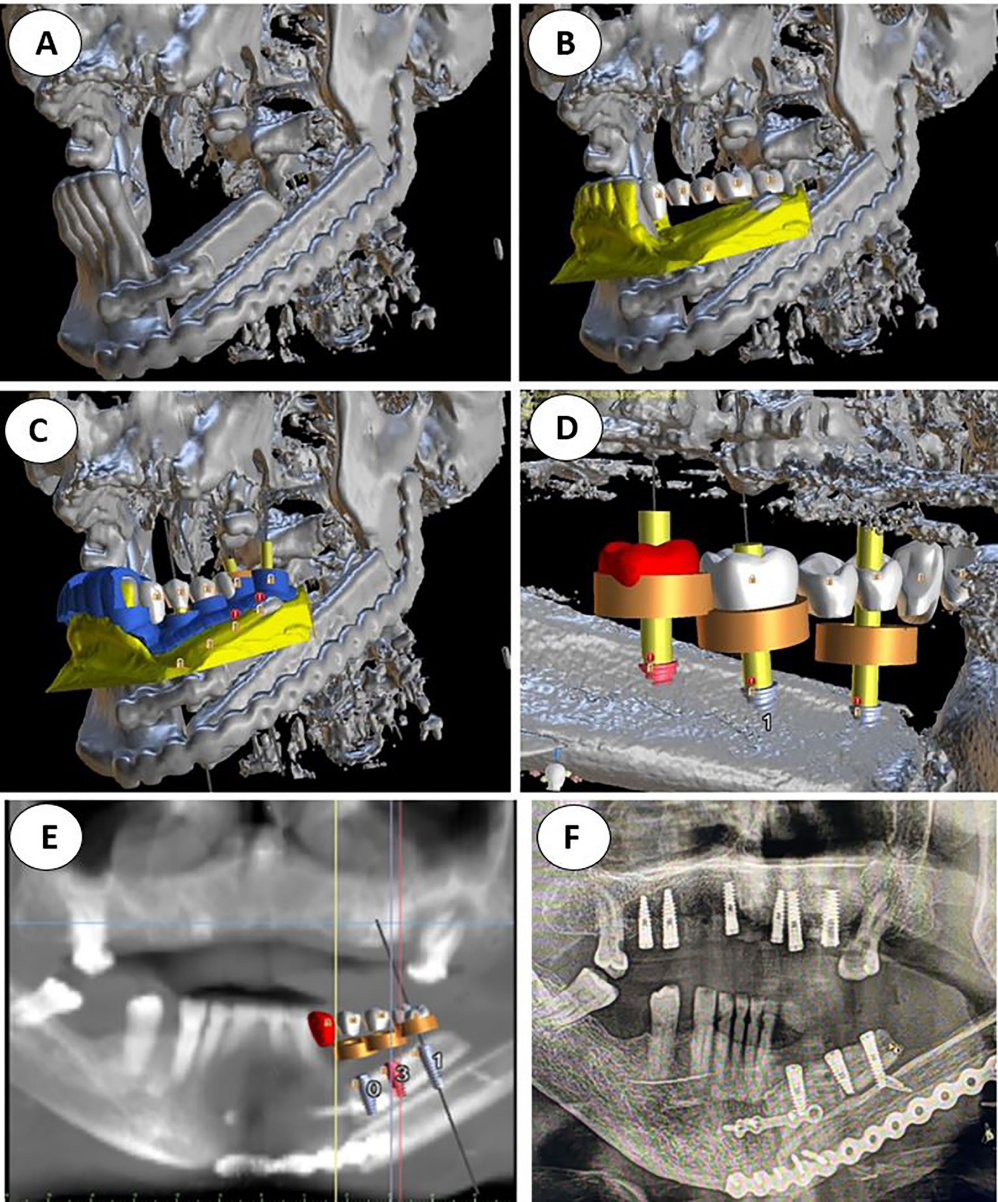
(A) Double barrel fibula flap CBCT 16 months after irradiation with 70 Gy. A basal reconstructive plate and a crestal miniplate. (B) Scanned lower jaw plaster model merged with the CBCT and virtual tooth design. (C) Prosthetically driven implant planning and in blue the teeth supported rigid splint designed with windows for insertion verification. (D) Lingual view seen from the floor of the mouth of the crestal fibula segment and the prosthetically driven implant placement. (E) VSP, preoperative implant planned position superimposed in the CBCT (F) Postoperative orthopantomogram.
